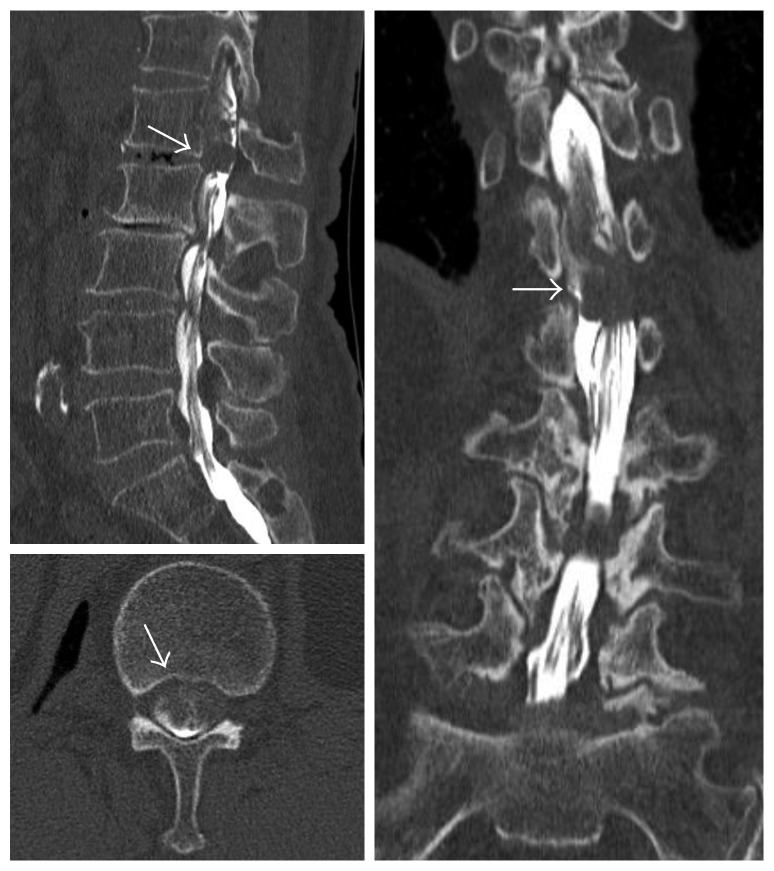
Computed tomographic myelography demonstrates the mass located in the right side of the intradural space, significantly compressing the cauda equina (arrow).
